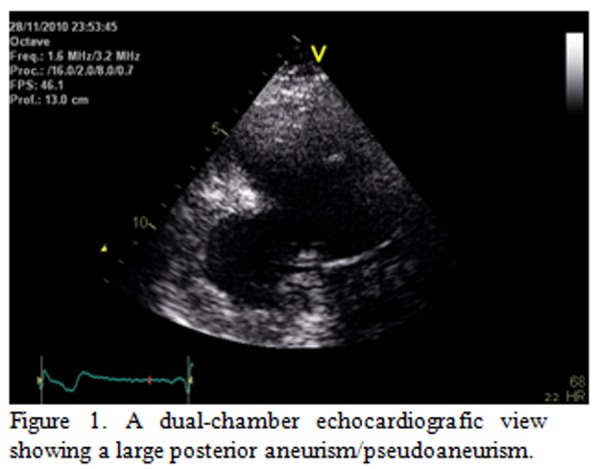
A dual-chamber echocardiografic view showing a large posterior aneurism/pseudoaneurism.
